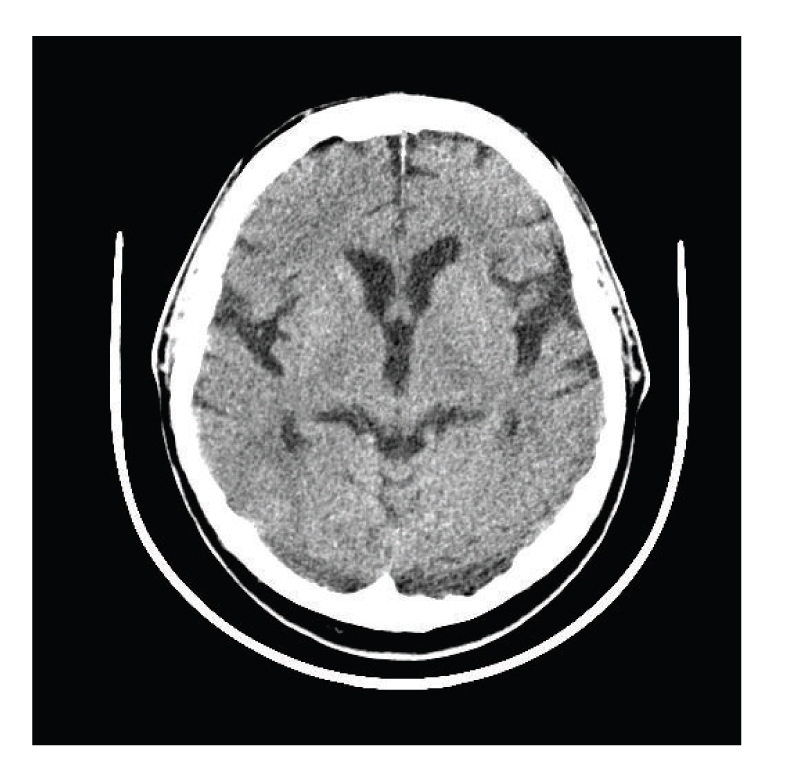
CT slice to extract ventricles.
